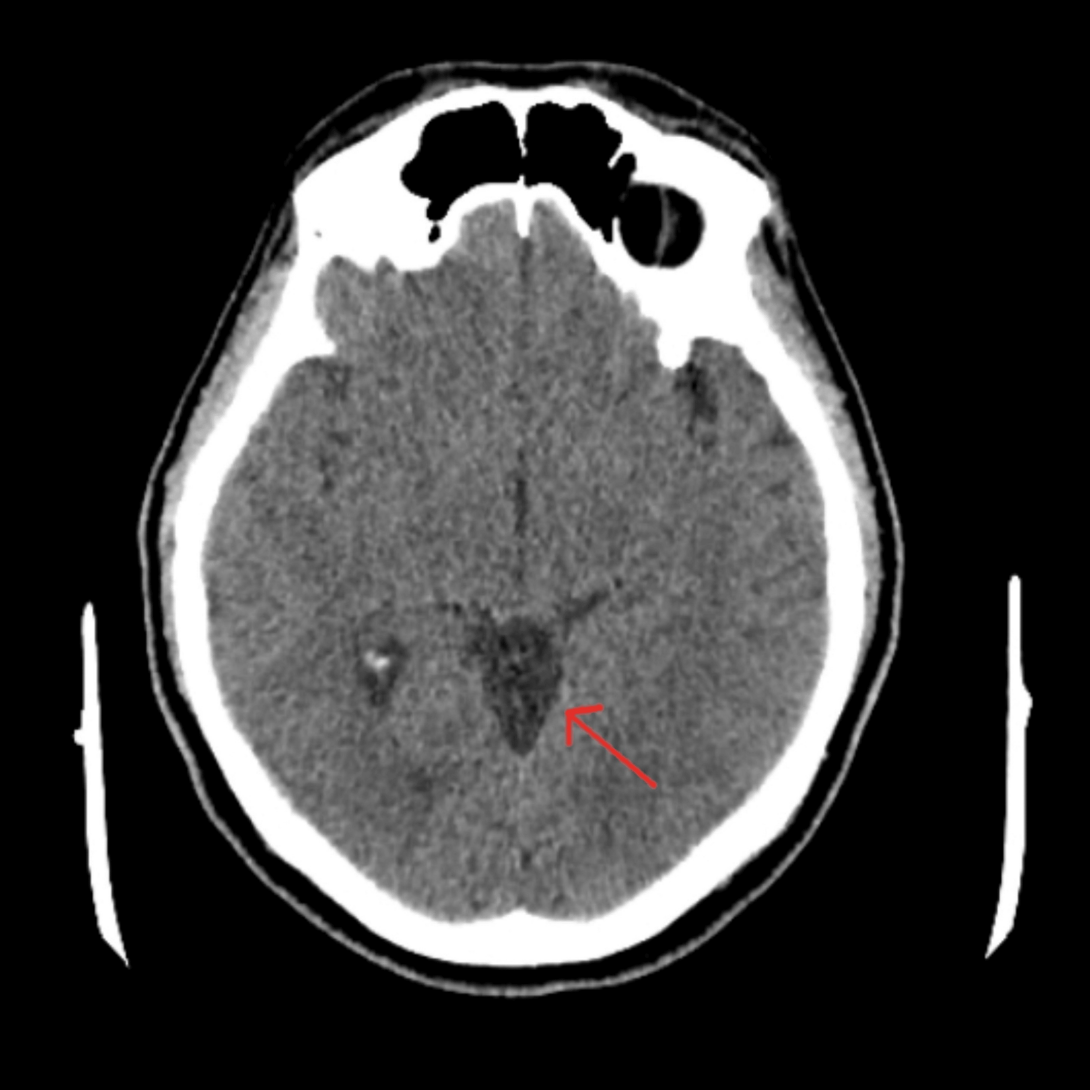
Axial non-contrast CT image demonstrating a well-defined, hypodense cerebrospinal fluid-attenuation lesion in the quadrigeminal cistern, consistent with an arachnoid cyst (arrow).
